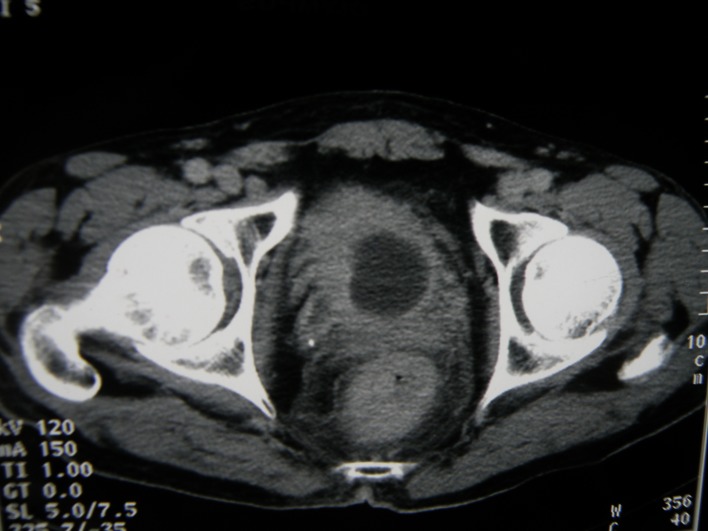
Computed tomography scan showing an important thickening of the bladder wall.
